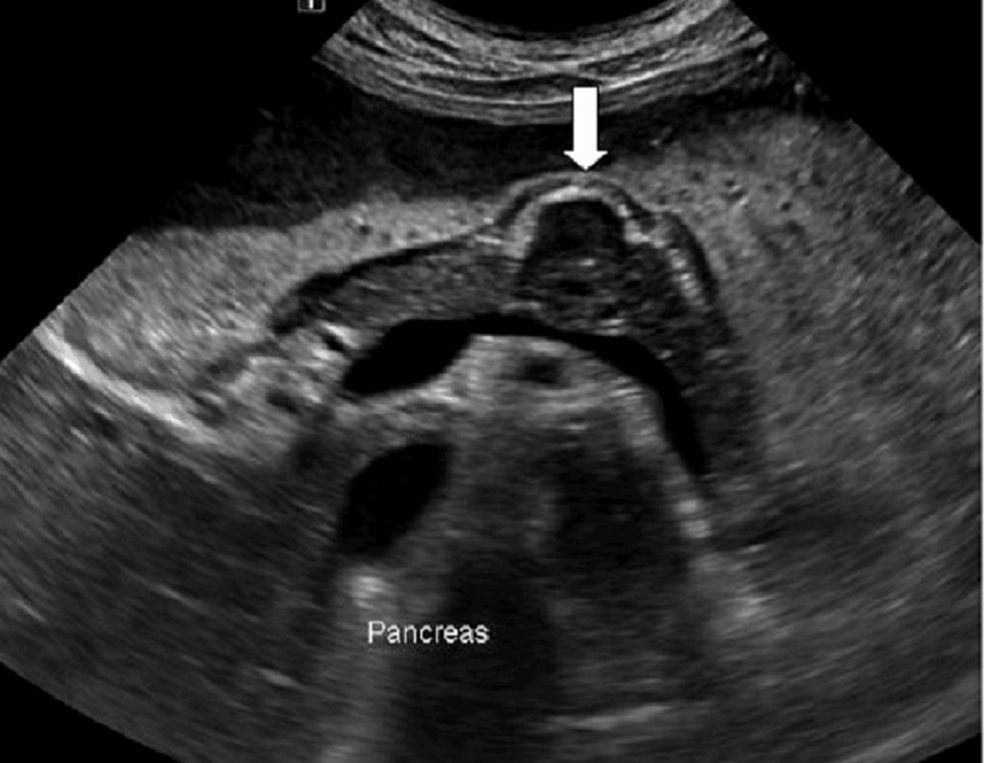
Ultrasound of the abdomen showing a distended stomach filled with fluid/food matter and hypoechoic lesion (white arrow) in the epigastric region related to the body of the pancreas
